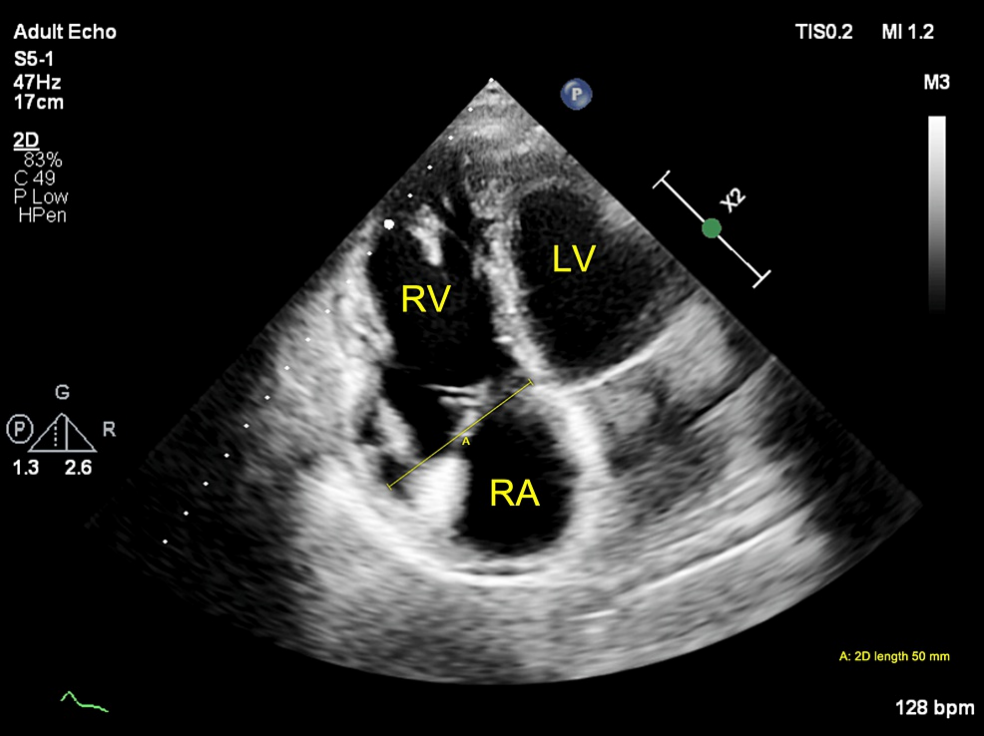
Two-dimensional TTE, apical 4-chamber view, diastolic phase, demonstrates a dilated RV with a basal diameter of 50 mm. TTE: Transthoracic echocardiography; RV: Right ventricle.
